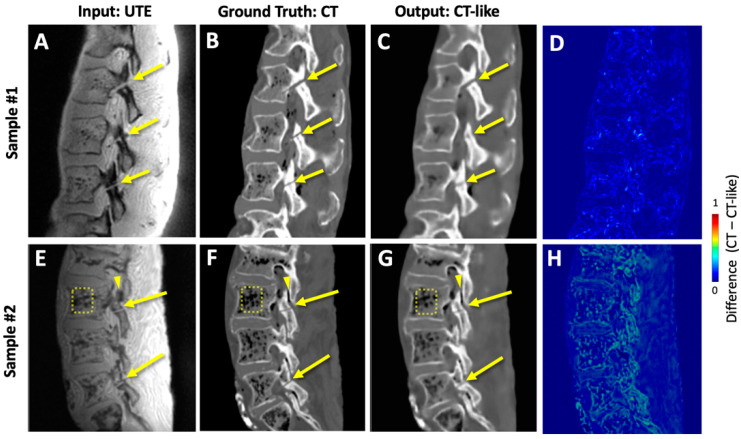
Results of image regression. UTE images (A,E) were used as input and trained on CT images (B,F) to synthesize CT-like images (C,G). Difference between CT and CT-like images is shown as color maps (D,H). Arrows indicate pars defects created experimentally. Compared to (E) UTE image that depicted both the bone and air in the vertebral body (square) and the facet joint (arrowhead) with low signal intensity, (F) CT-like image correctly depicted the bony structures with high signal intensity and the air with low signal intensity. The overall correspondence between CT-like and CT images was excellent, but CT-like images were not as sharp.
